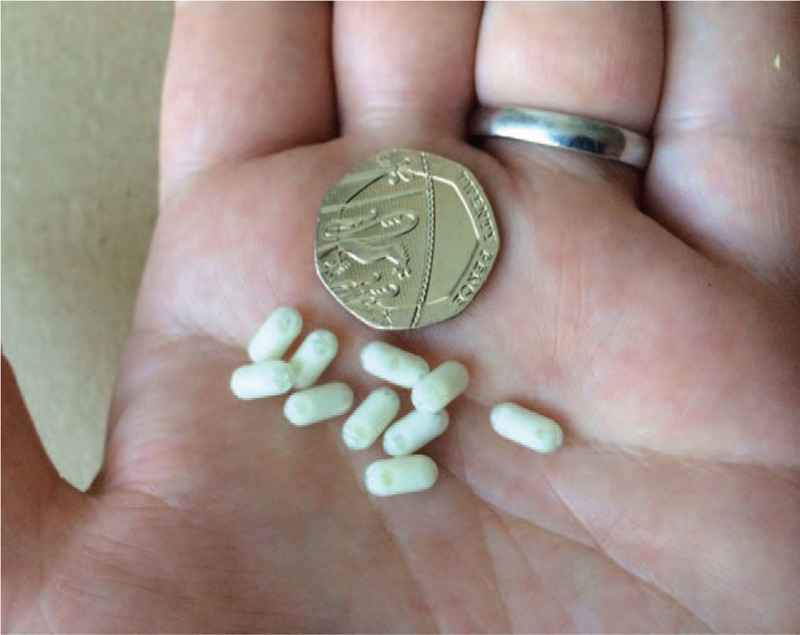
Mini-capsule MRI device markers pictured near a 20 pence coin (21 mm in diameter) for size comparison.
